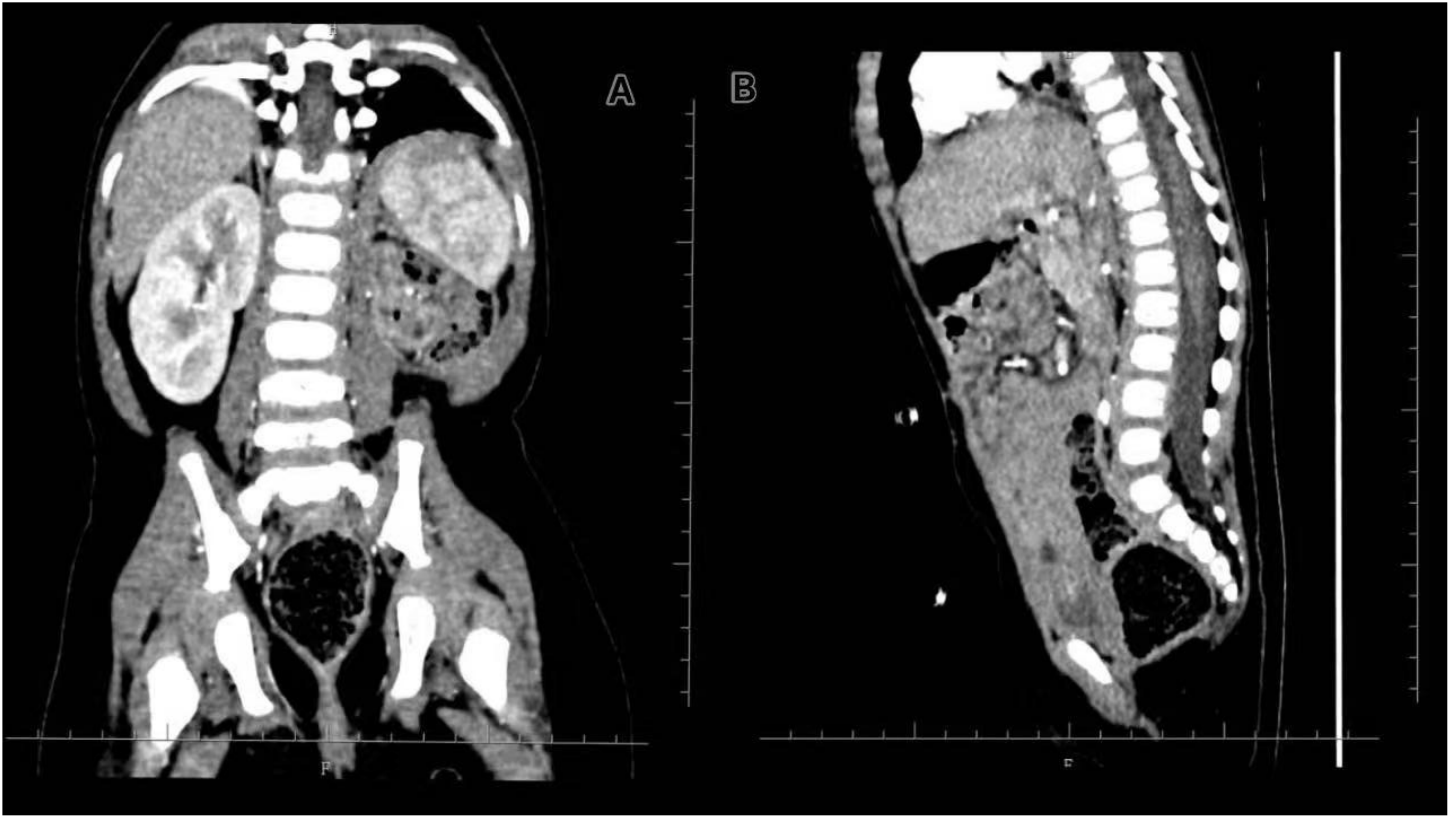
Follow-up CT to assess vertebral body growth after proton beam therapy (PBT). (A) Coronal reconstruction demonstrating spinal alignment and symmetry. No significant scoliosis or vertebral wedging is observed. (B) Sagittal reconstruction demonstrating vertebral height and spinal curvature.
